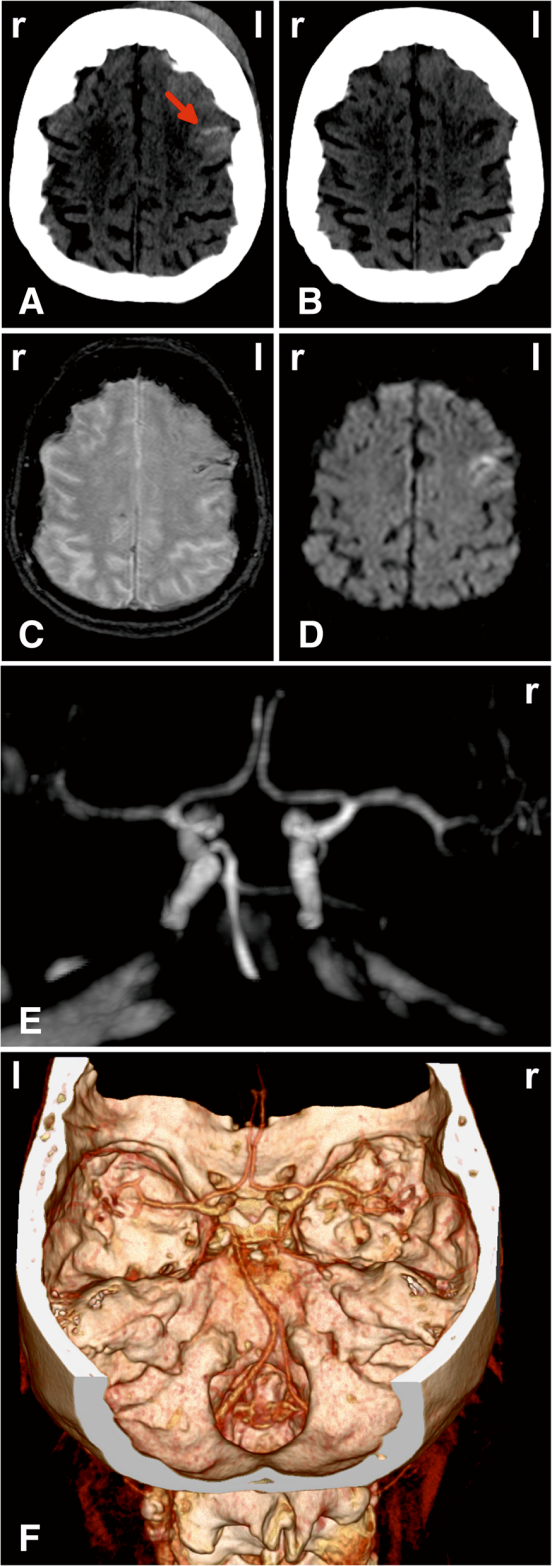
Development of a cortical infarct around sulci filled with subarachnoid blood. (a) is a representative image of the initial CT scan on day 0. It shows subarachnoid blood in two sulci of the left frontal cortex (arrow). In addition, a left-sided hematoma exterior of the head marks the area of the impact. (b) Whereas the subsequent CT scans on days 1, 3, 5 and 9 showed no new infarcts, the follow-up CT scan on day 14 revealed a new hypodensity confined to the cortical gray matter around the sulci that had shown subarachnoid blood in the CT scan on day 0. This lesion was consistent with the new infarct seen on the MRI scan of day 10. (c) The T2* weighted image of the MRI on day 10 revealed that the subarachnoid blood was still present in the sulci in which it had been seen on the initial CT scan, although the hyperdensity had disappeared on later CT scans. (d) The DWI on day 10 showed a hyperintensity typical of a new infarct around the sulci with subarachnoid blood. This DWI hyperintensity corresponded to an ADC reduction (not shown). The synopsis of images (a) – (d) provides evidence for delayed cortical laminar necrosis adjacent to the sulcal clot in the left frontal cortex. (e) The MRA on day 10 and (f) the CTA on day 14 did not show any evidence of proximal vasospasm
